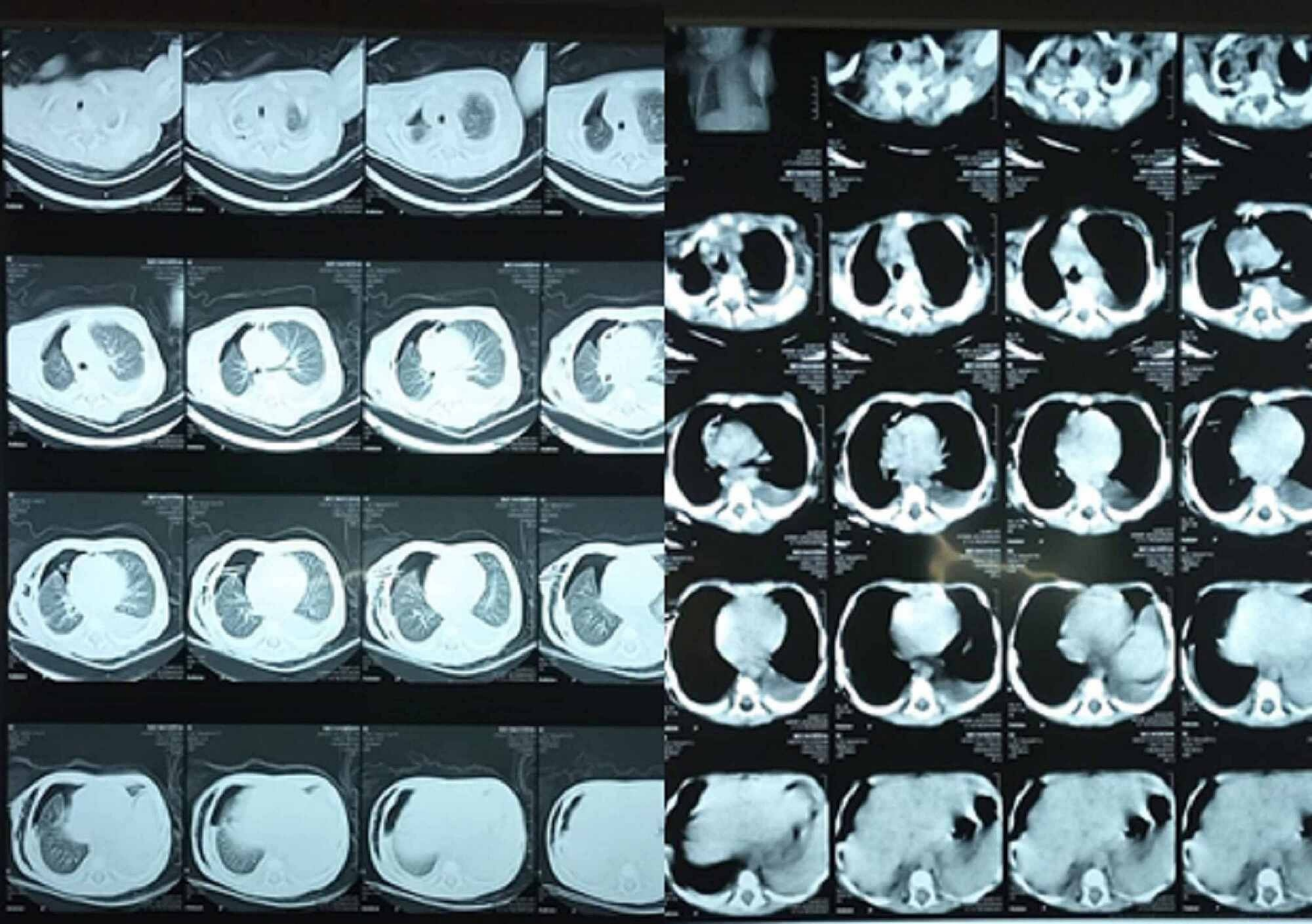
Computed tomography chest showing bilateral pleural effusion, subcutaneous emphysema, and pneumothorax.
